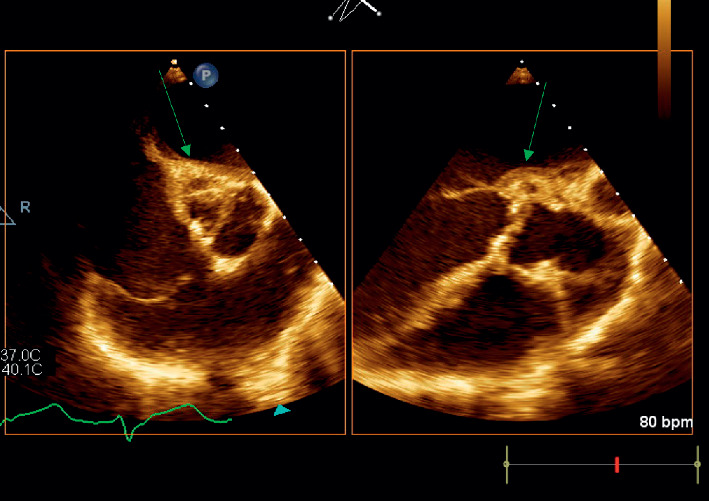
Transesophageal short axis and long axis view, respectively, of the aortic valve showing multiple echolucent areas of the aortic root suggestive of aortic root abscess.
